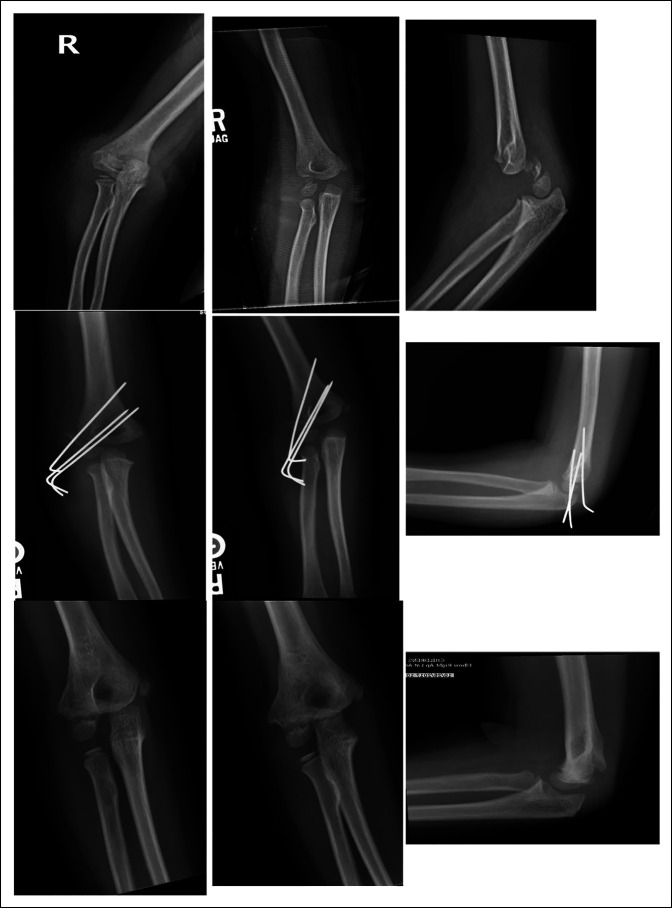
Three-year-old girl: A–C, AP, oblique, and lateral radiographs of initial injury. D and E, Three weeks post-op. F–H, Eleven months post-op with osteophyte formation posterior and laterally.
